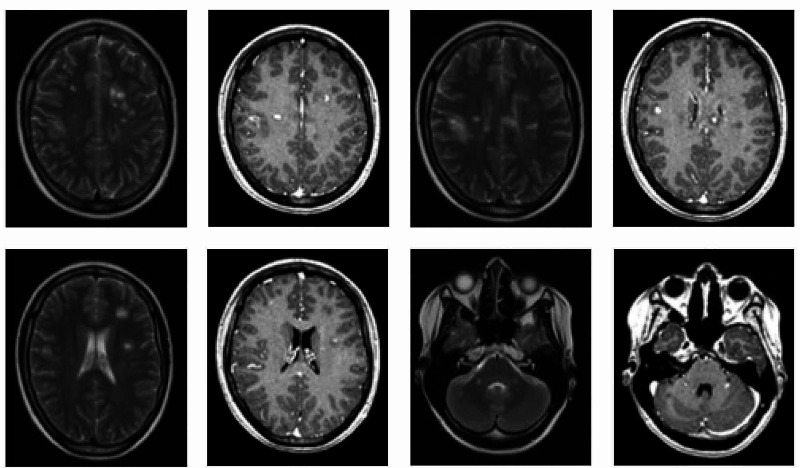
MRI brain - four weeks post injury; T2 weighted and T1 weighted with contrast at different axial cuts
